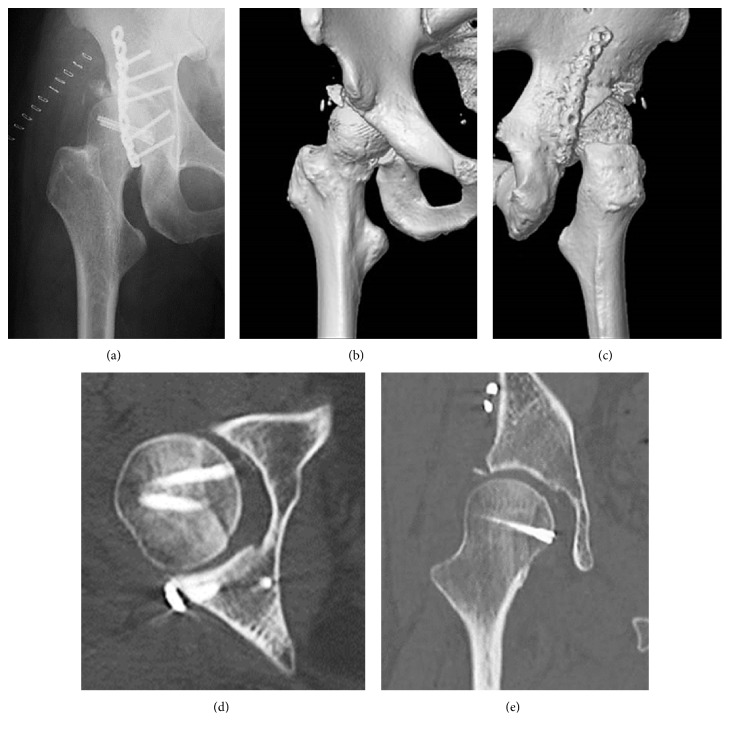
Case 1. (a) Anteroposterior X-ray image of the right hip after open reduction and internal fixation. (b) Anteroposterior three-dimensional computed tomography showing acceptable reduction of the femoral head. (c) Posteroanterior three-dimensional computed tomography showing acceptable reduction of the posterosuperior wall. (d) Axial computed tomography showing acceptable internal fixation of the femoral head and posterior wall. (e) Coronal computed tomography showing acceptable internal fixation of the femoral head and congruity.
